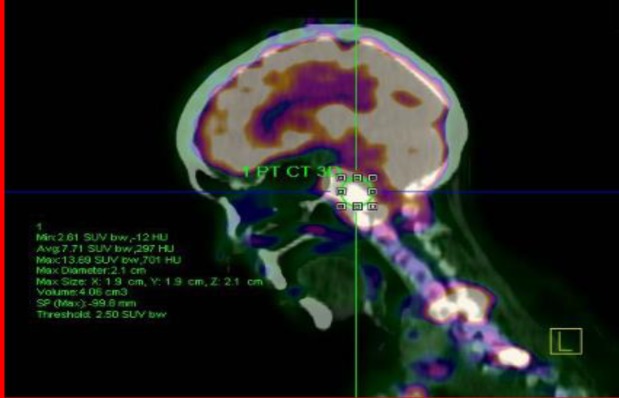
Sagittal reconstructed 18F-FDG PET-CT showing multiple bony involvement ,with the skull base including the right petrous bonebone with intracranial extension and multilevel axial skeleton involvement including the upper cervical spine (C1 to C4), dorsal vertebral (D11)
